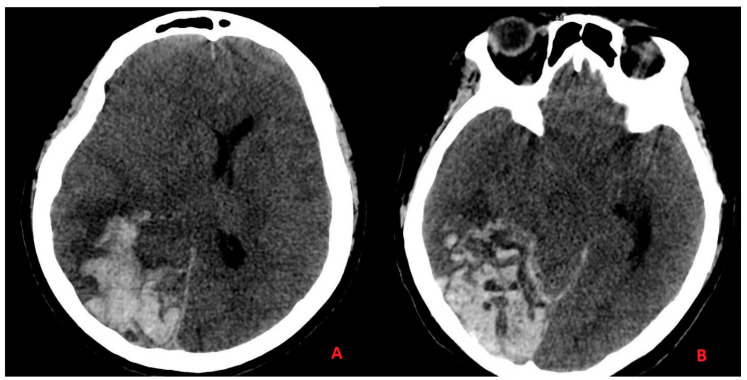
Acute ischemia with hemorrhagic infarction in the absence of any vascular malformation with acute quote of cerebral edema showed on CT head scan images. In panel (A), we can see the hemorrhagic infarction of the parietal and temporal lobes, with edema on the white matter of the antero-lateral portion of the temporal lobe with added “mass effect” on the adjacent structures. In panel (B), we can see the hemorrhagic infarction of the occipital and temporal lobes, and the subarachnoid hemorrhage on the cerebellar tentorium margin.
